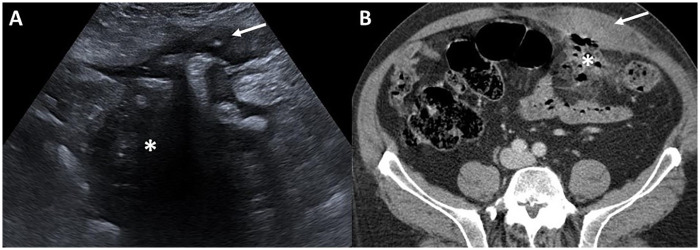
Acute left colonic diverticulitis stage 2 in a patient with previous episodes of diverticulitis. At the time of exploration, a US image obtained with a convex transducer (A) shows a locally liquid collection (*) >4 cm close to the colonic wall that seems extend to the abdominal wall. A contrast-enhanced CT in the axial plane (B) shows part of the abscess with gas (*) and an inflamed left rectal abdominal muscle (white arrow).
